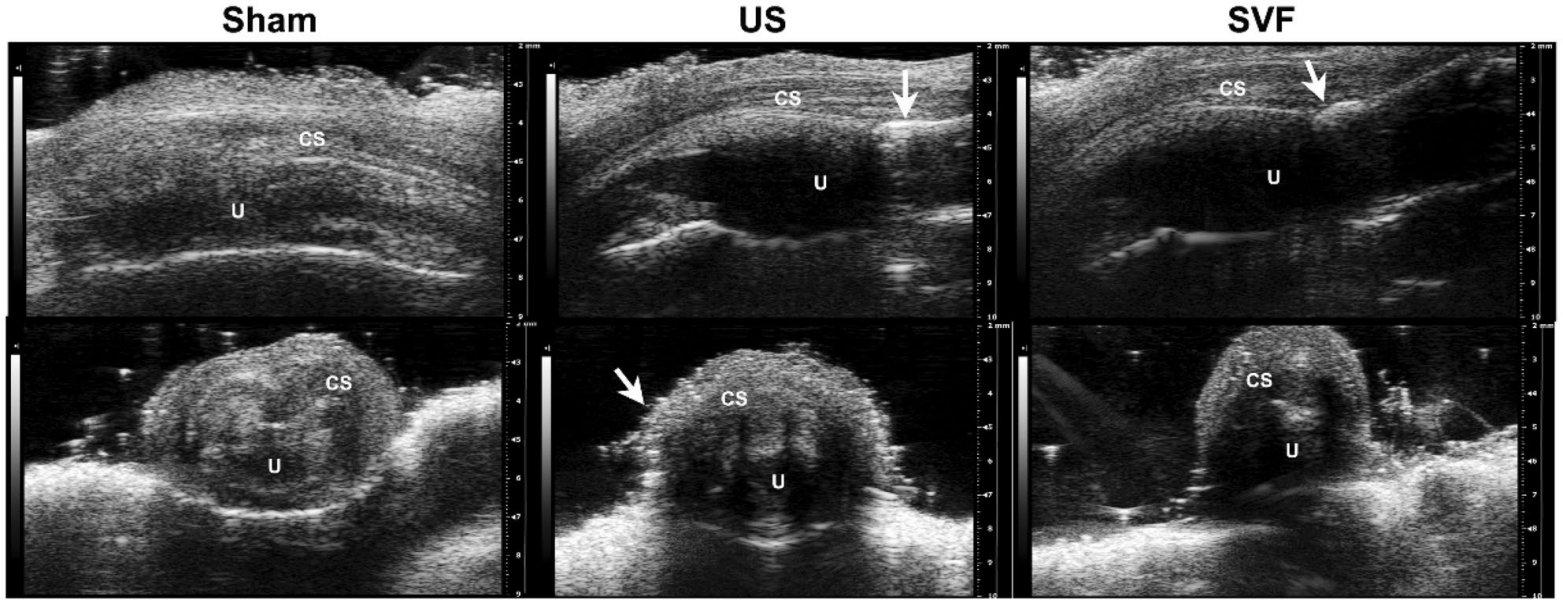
Representative microultrasound images of penile urethras in sham, US and SVF groups at 4 weeks after surgery. White arrows indicate sites of hyperechogenic tissues and narrowing of the urethral lumen in the US and SVF groups. *CS* corpus spongiosun, *U* urethral lumen.
